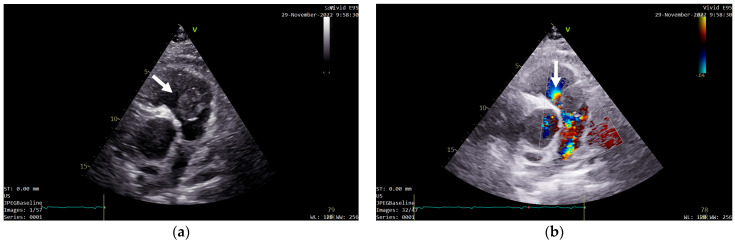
Transthoracic echocardiography (TTE) showing: (a) a round intracardiac mass attached to the right ventricle outflow tract (RVOT) wall by a pedunculus (white arrow); (b) turbulent flow on Color Doppler caused by the tumor’s severe obstruction of the RVOT (white arrow).
